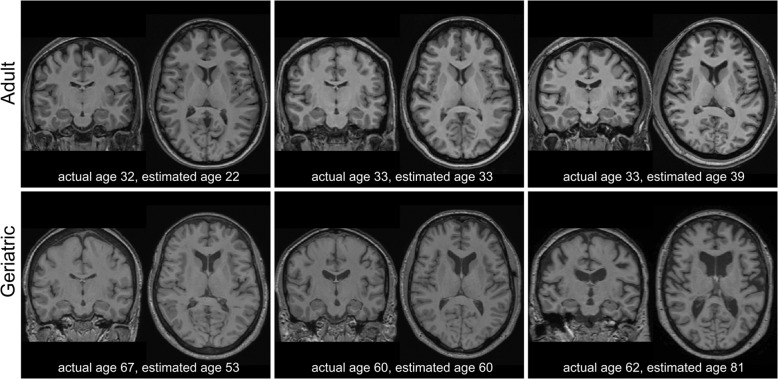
Comparison of structural MRI of participant brains in midlife and older adult cohorts. Each image is a separate participant, displaying coronal and axial images and actual (chronological) age and estimated (calculated) age. The top row is from the midlife adult cohort and the bottom row is from the geriatric cohort.
